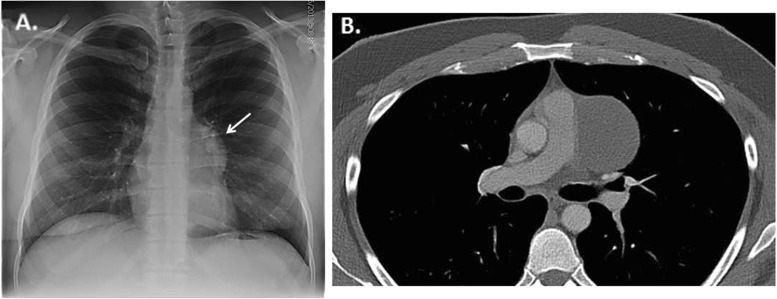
Teratoma. a. Posteroanterior chest radiograph demonstrating a mass in the aortopulmonary window (arrow). b. Contrast non-gated axial CT image demonstrating a cystic pericardial mass. Subsequent cardiac MR suggested possible solid components atypical for a simple pericardial cysts. Pathological analysis post-resection identified the mass as a teratoma
